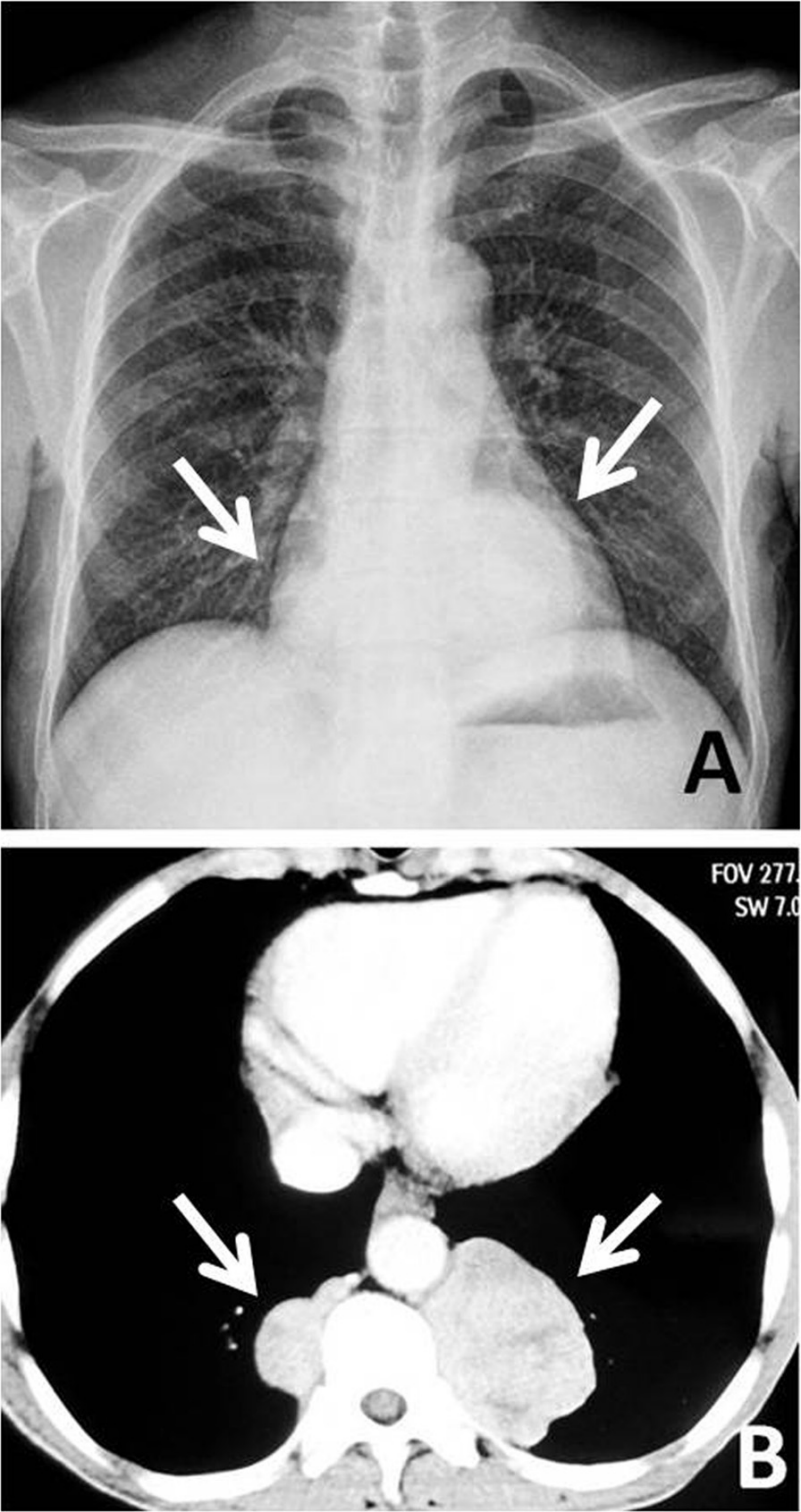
Chest X-ray and contrast-enhanced CT features of the case. A: Posteroanterior chest X-ray showing a bilateral mass in the posterior mediastinum. B: Contrast-enhanced CT scan showing a bilateral thoracic paravertebral mass.
